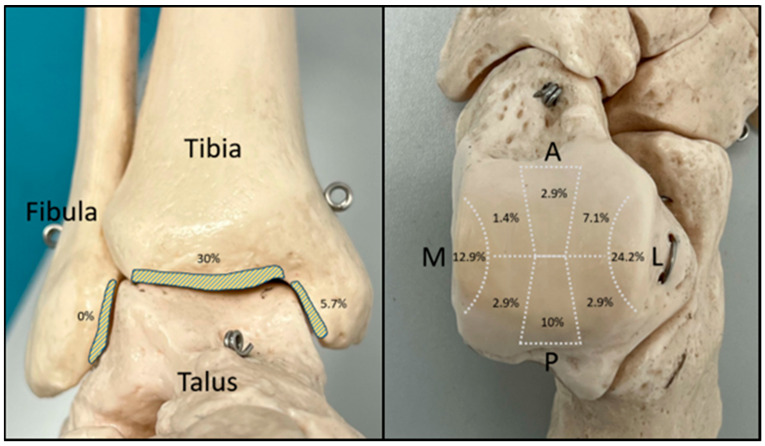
Zone allocation of the detected CLs in the preoperative MRI (M, medial; L, lateral; A, anterior; P, posterior).
